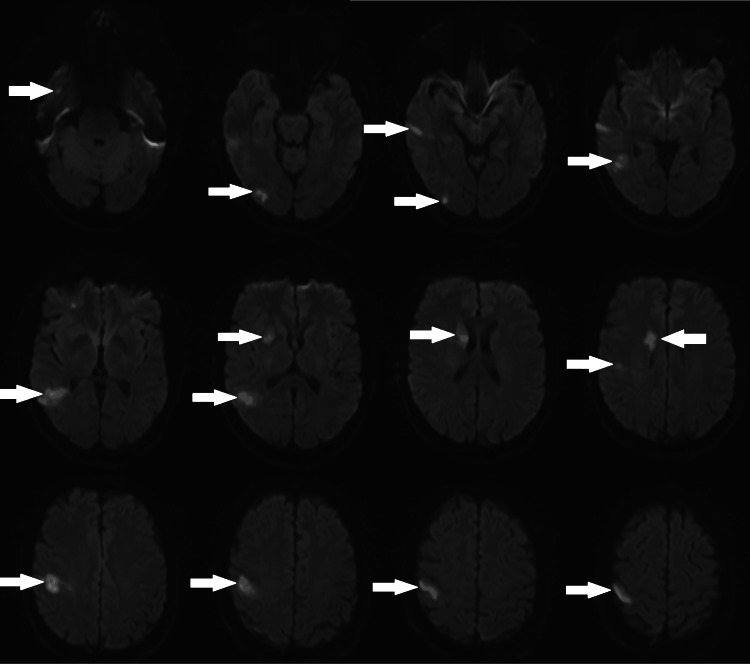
Axial MRI DWI baseline with arrows showing the initial right anterior circulation infarct. MRI DWI, Magnetic Resonance Imaging Diffusion-Weighted Imaging
